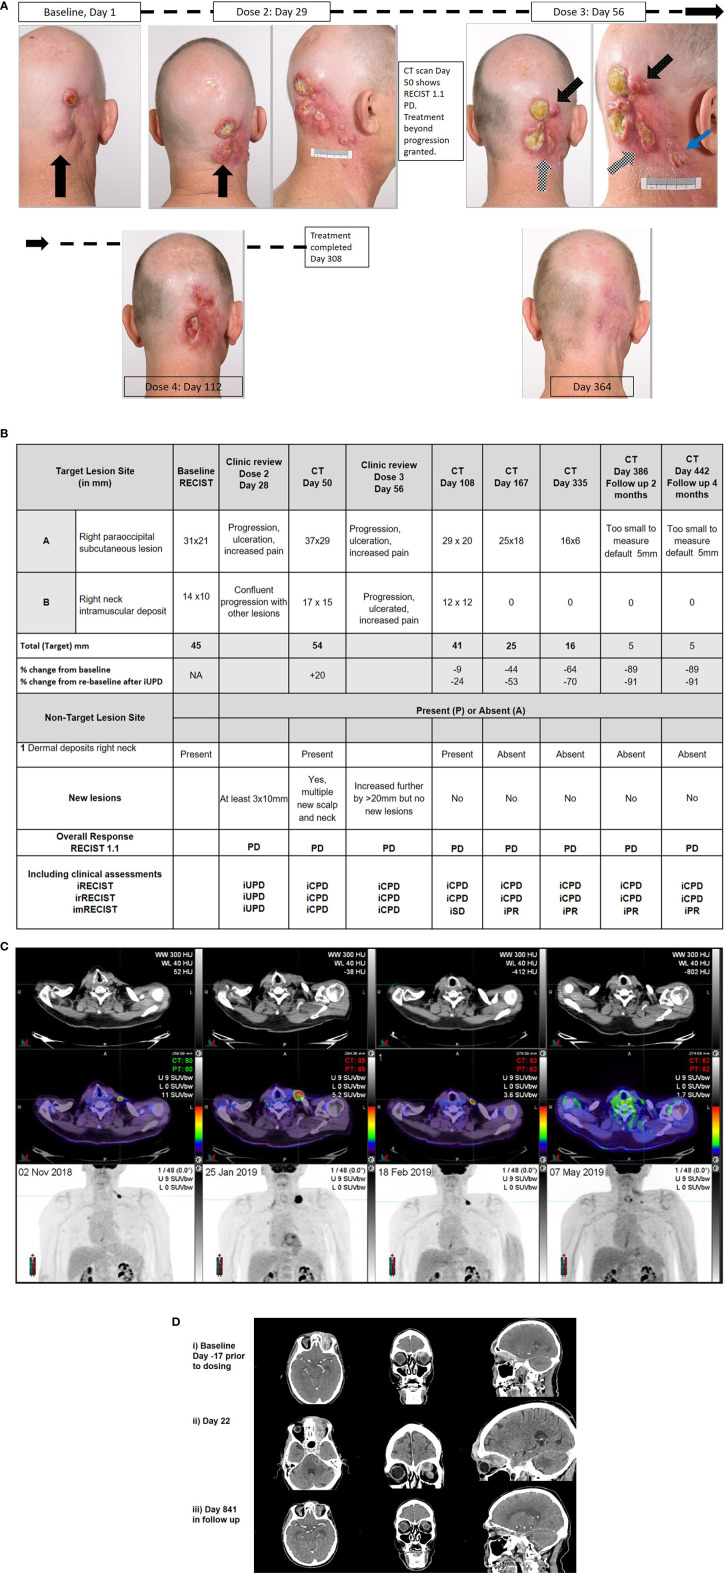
Correlative clinical photographs, tumor response measurements according to time, and fluorodeoxyglucose - positron emission tomography (FDG-PET) and CT scan images of discussed patient cases. (A, B) Patient One’s photographic images demonstrating iUPD and iCPD, with accompanying tumor response measurements according to RECIST 1.1 and immune based criteria at key time points. (C) Patient Two’s FDG-PET images over time with low dose axial CT, fused axial CT, and maximal intensity projection (MIP) images in each column from 02/NOV/2018, 25/JAN/2019, 18/FEB/2019, and 07/MAY/2019, demonstrating evidence of progression and regression with ongoing immunotherapy. (D) Patient Three’s representative images of disease at baseline, at progression and with response. Images i) Baseline (Day-17 prior to dosing) Post contrast CT: (axial, coronal, sagittal) demonstrates enhancing soft tissue extending from the cutaneous left forehead along the roof of the left orbit in the distribution of V1, involving the cavernous sinus, Meckel’s cave, nerve root entry zone of the left trigeminal nerve and likely involvement of the trigeminal nuclei with soft tissue at the left pons. ii) Day 22 Post contrast CT: (axial, coronal, sagittal) demonstrates increasing volume of enhancing soft tissue extending from the cutaneous left forehead along the roof of the left orbit in the distribution of V1, involving the cavernous sinus, Meckel’s cave, nerve root entry zone of the left trigeminal nerve and involvement of the trigeminal nuclei with soft tissue at the left pons. iii) Day 841 (in follow up) Post contrast CT: (axial, coronal, sagittal) demonstrates small volume residual non-enhancing soft tissue extending from the cutaneous left forehead along the roof of the left orbit. The remaining soft tissue has resolved on CT.
